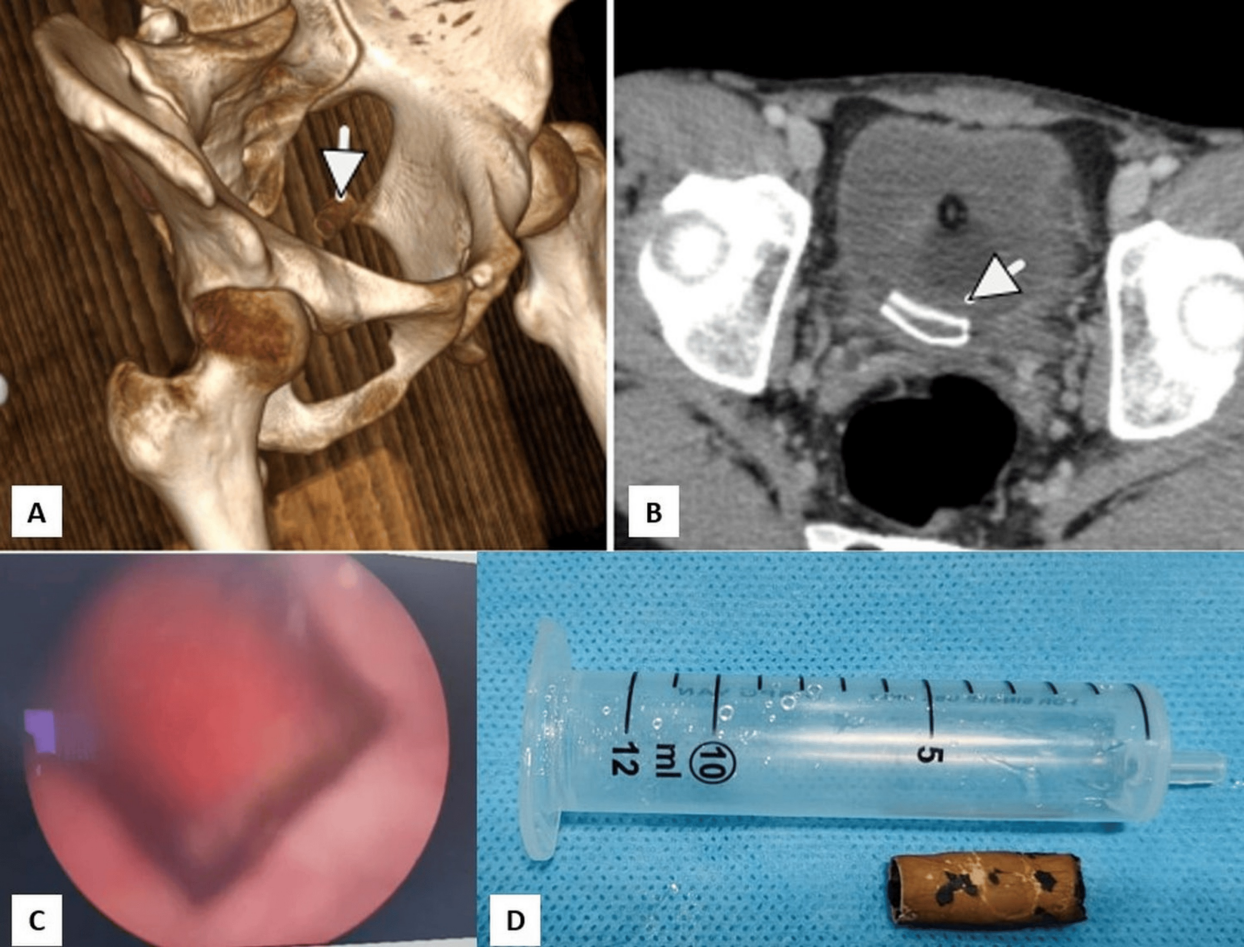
Imaging and retrieval of self-inserted urethral foreign body (Case 1). (A) 3D reconstructed CT scan showing a tubular foreign body within the bladder (white arrowhead). (B) Axial CT scan demonstrating encrustation over the foreign body (white arrowhead). (C) Cystoscopic view of the foreign object within the bladder. (D) Retrieved electrical rubber casing placed adjacent to a 10 mL syringe for scale comparison.
